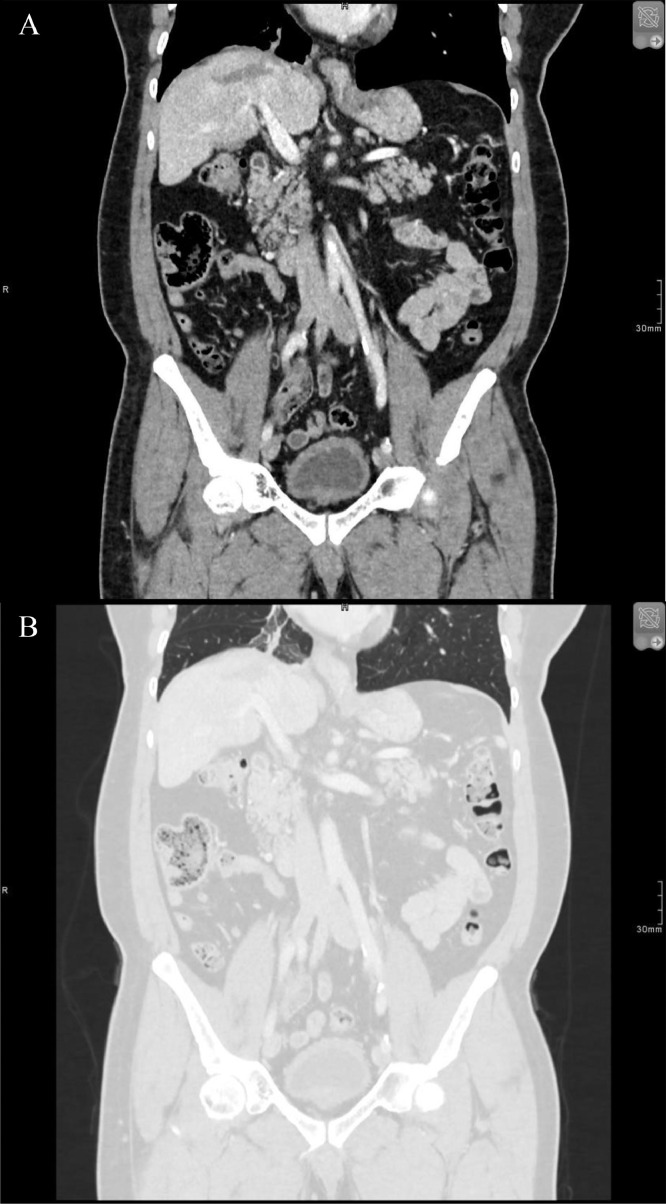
Follow-up enhanced CT abdomen. Coronal soft tissue (A) and lung window (B) images. Regression of the pleural components of the fistula tract and the right lower lobe consolidation with small residual atelectasis and ground-glass opacity.
